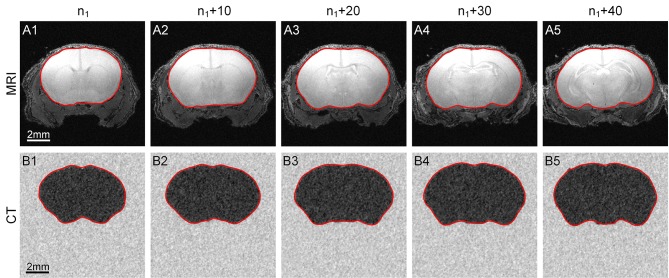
Segmentation of MRI and CT data. The results obtained by applying our method to MRI and CT data. (A1–A5) The 1st row shows the contour evolution process in MR images. (B1–B5) The 2nd row shows the process using CT data. The red curves are the computed CCS of the outline of the target area. n_1_ represents the 10th section of the image sequence of every dataset. The image to the right of each column is the 10th image after it.
